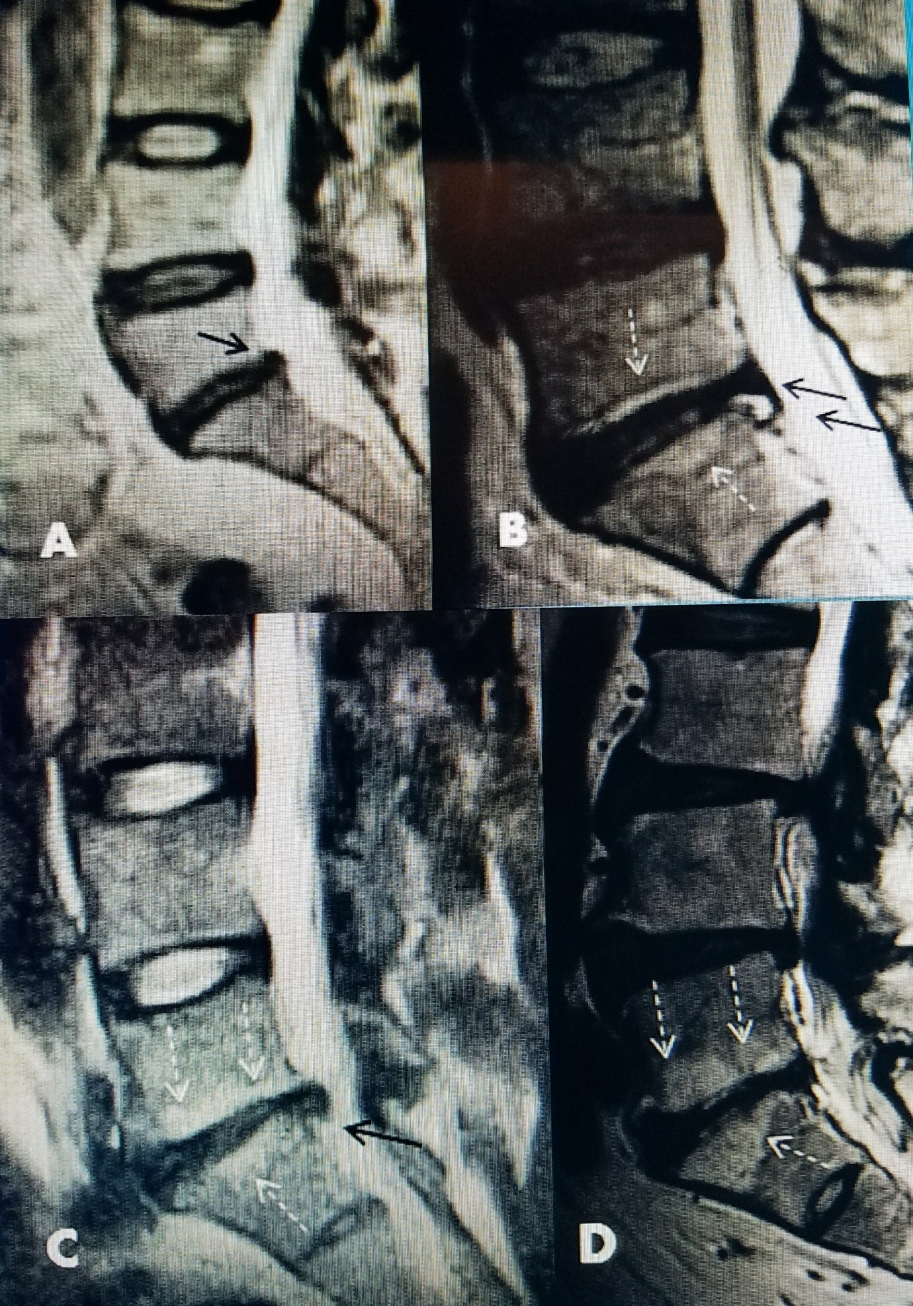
Examples of L5-S1 disc degeneration A: Sagittal T2 magnetic resonance imaging (MRI) showing small posterior herniation (black arrow) with some T2 signal change in both L5-S1 and L4-5 disc. B: Mild narrowing L5-S1 disc with small subligamentous herniation (solid black arrow) and bulging thickened annulus above (dotted arrow). There is very early minor endplate Modic change in middle of L5-S1 endplates (dotted white arrow). C: Progressive narrowing at L5-S1 with annular bulge rather than disc herniation (black arrow) and more marked Modic changes in endplate (dotted white arrows). D: More severe Modic change (dotted white arrows) with no evidence of disc or annular herniation. There is associated stenosis at L4-5 and L3-4 that is above the L5-S1 degenerative disc.
